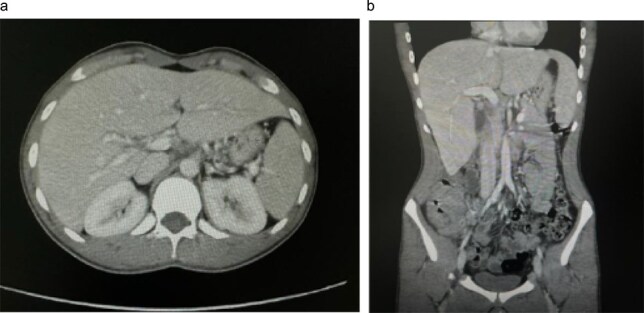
Initial contrast-enhanced computed tomography (CT) abdomen. (a) Axial image demonstrating a 3.8 × 3.3 cm lesion in hepatic segment 4B, suspicious for metastasis. (b) Coronal reformatted image showing the hepatic lesion in association with right-sided colonic wall thickening, raising initial concern for primary colonic malignancy with hepatic metastasis.
